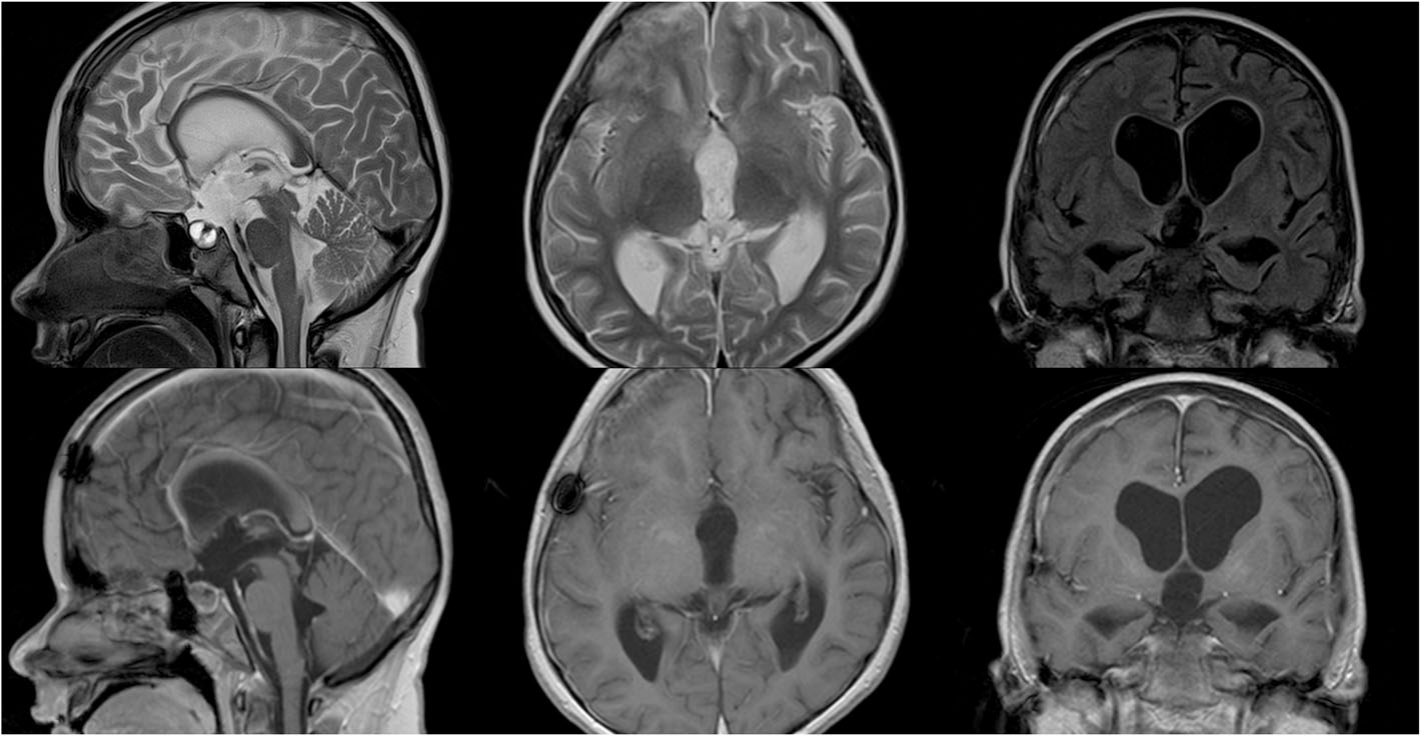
Control magnetic resonance imaging scan (carried out on day 152 after surgery)
